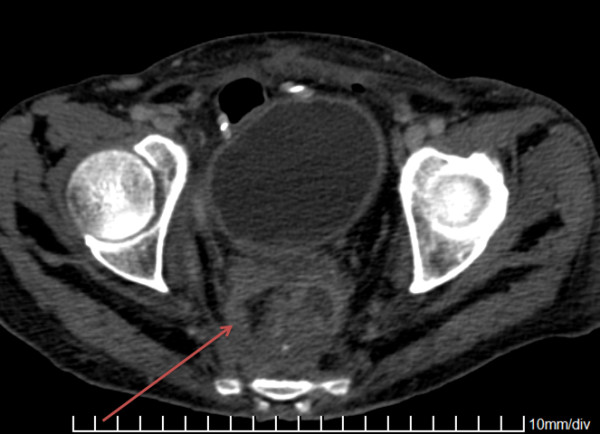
Computed Tomography scan of pelvis showed rectal abscess (arrowhead).
